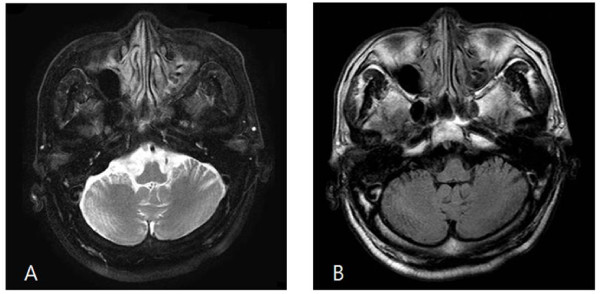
A follow-up MRI three months after discontinuation of metronidazole shows complete resolution of the previously noted signal changes. A: T2-weighted image. B: FLAIR image.
